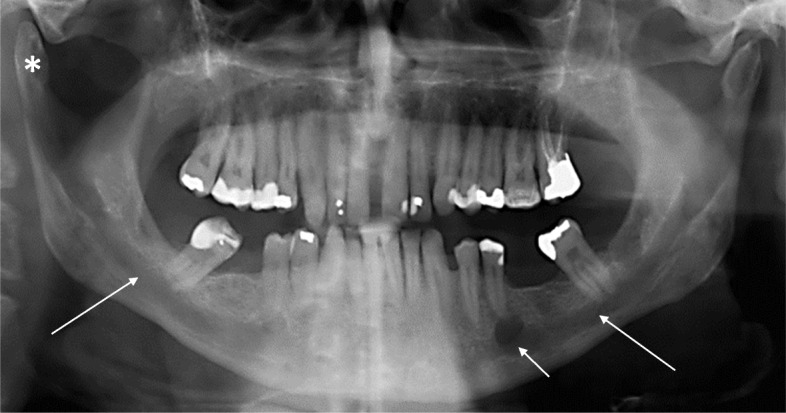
Orthopantomogram of a 55-year-old man with NF1. The mandibular canals (long arrows) on both sides of the mandible are widened in their full length and the mental foramen (short arrow) on the left side is enlarged. Note also the elongated right mandibular condyle (*)
